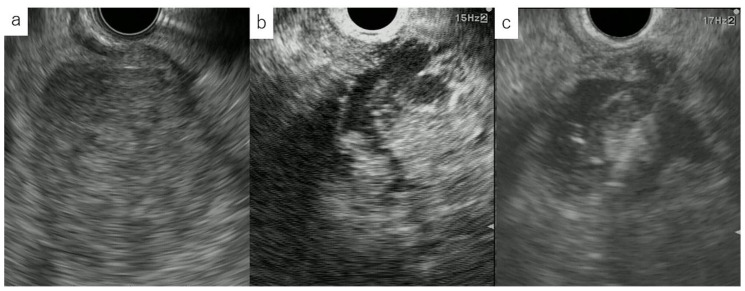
Targeting EUS-FNA with CH-EUS. (a) Fundamental B mode endoscopic ultrasonogram showing a low echoic mass. (b) CH-EUS image showing a non-enhancing area within the tumor. (c) EUS-FNA with CH-EUS avoiding the non-enhancing area.
